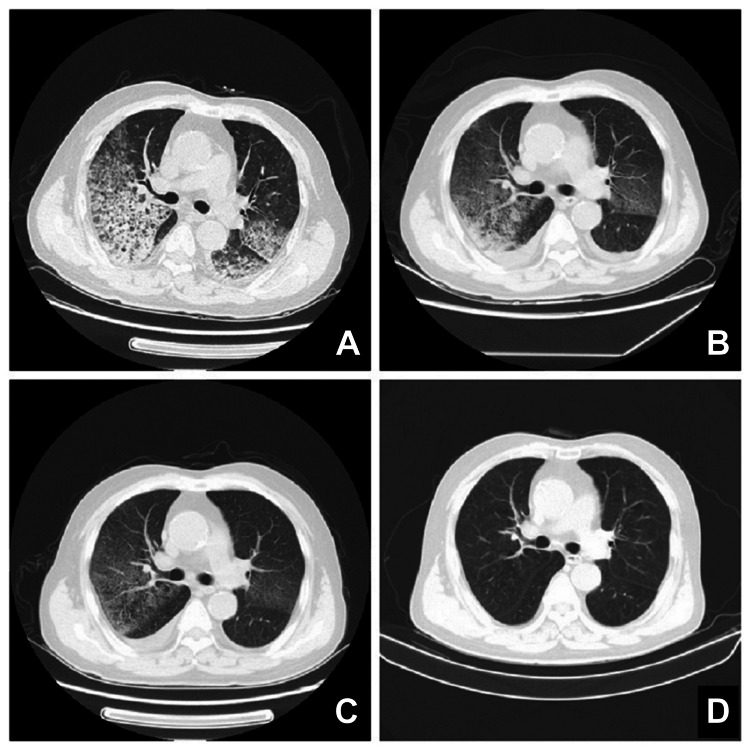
Serial chest computed tomography (CT) scans of a 66-year-old man with acute myocardial infarction. (A) Widespread new bilateral alveolar infiltrates 22 hours after tirofiban administration (diagnosis of DAH). (B) Reduced bilateral infiltrates on day five after tirofiban discontinuation. (C) Further resolution of infiltrates on day 10. (D) Complete clearance of infiltrates on day 15.
